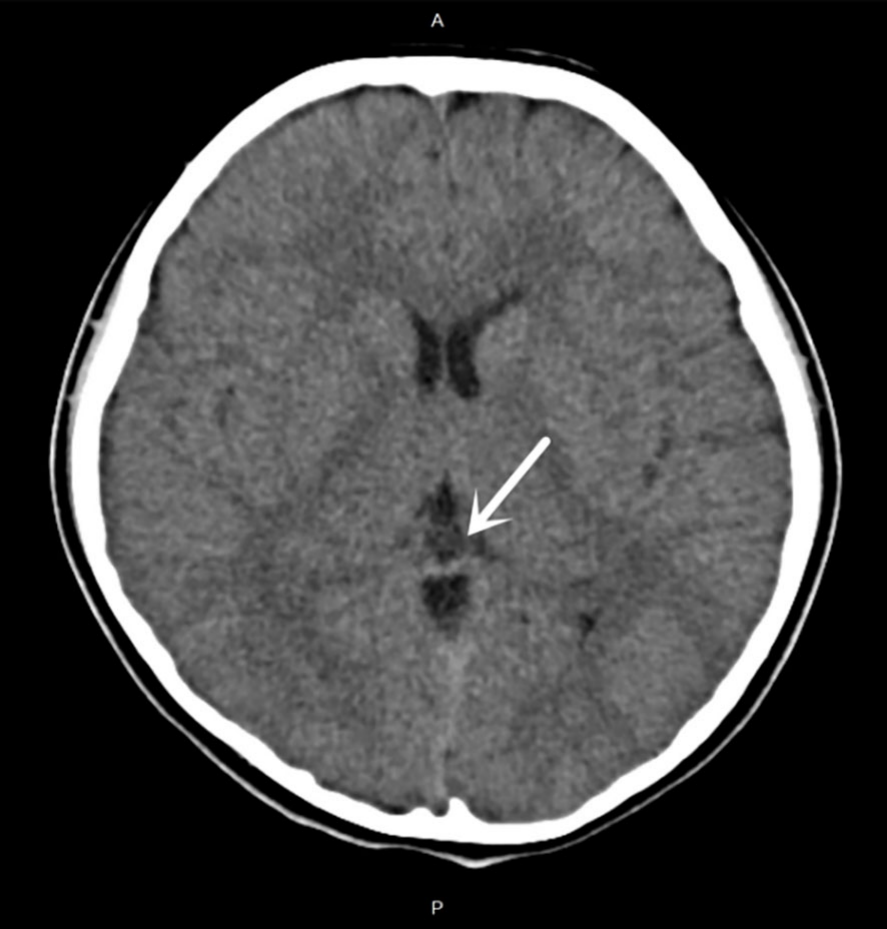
Non-contrast cranial CT showing a well-circumscribed, near-circular, water-attenuation cystic lesion located in the pineal region, consistent with a pineal cyst. White arrows indicate the pineal cyst location in axial CT image.
